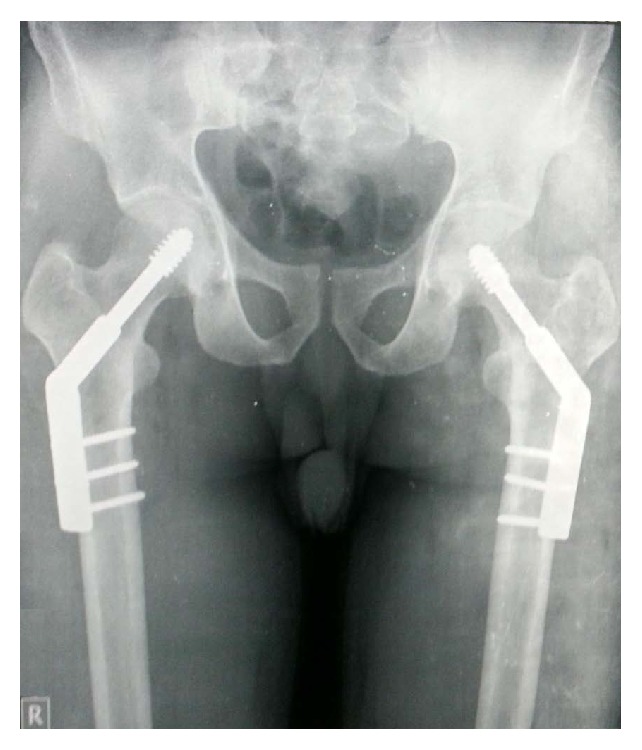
6 months postoperative X-ray of bilateral hip joint with pelvis showing 3 holed dynamic hip screw implant in situ with no articular changes.
